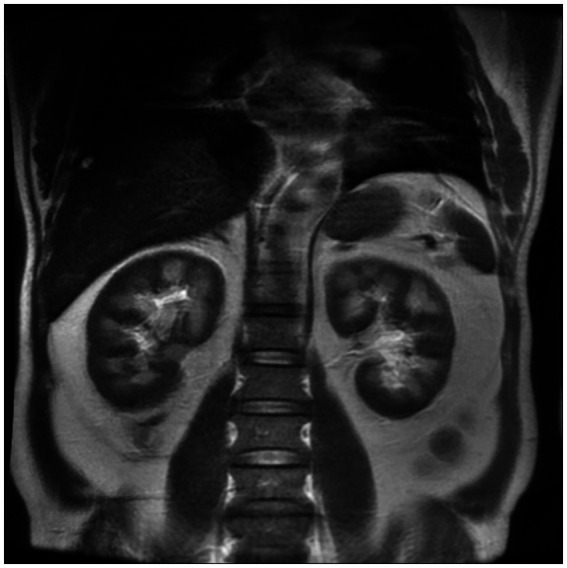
Renal magnetic resonance imaging. The renal MRI showed that the signal of the cortex on T1WI was lower than that of the medulla, showing a typical reversal of the renal cortex and medulla, suggesting that the cortex had manifestations of hemosiderin deposition.
